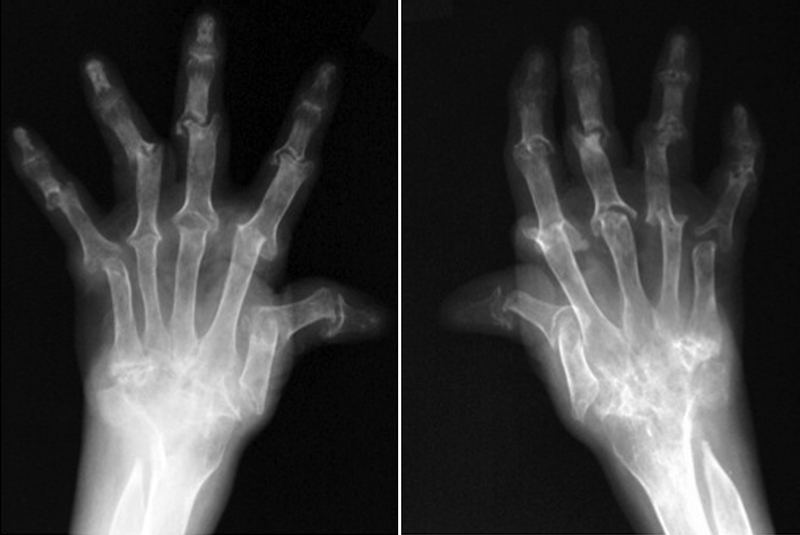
X-ray of both hands showed typical mutilating changes.
